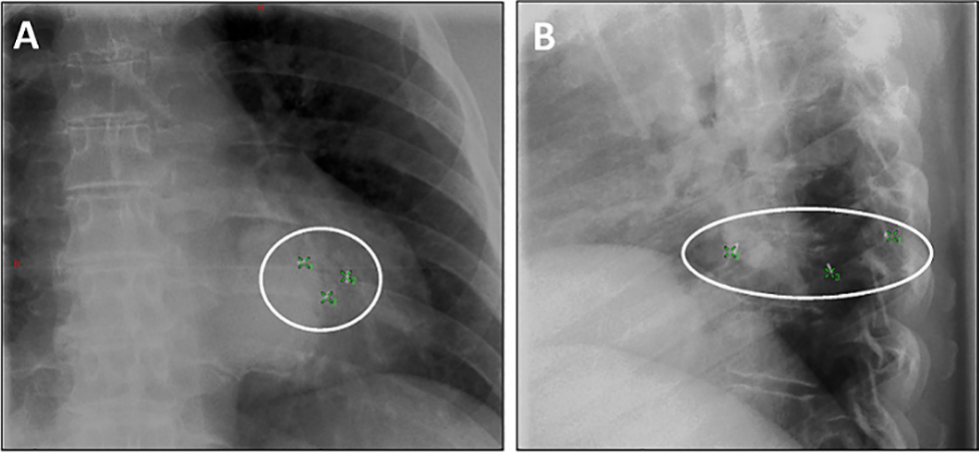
Position of three SuperLock nitinol coil fiducials used in two dimensional (2D) marker matching just prior to SBRT treatment for a left lower lobe lung tumor as visualized using on-board kV imaging (panel A: anterior-posterior image; panel B: lateral image). Three green crosses showed locations of the fiducials identified from the planning CT. The background images were 2D kV-kV pair images acquired prior to treatment.
